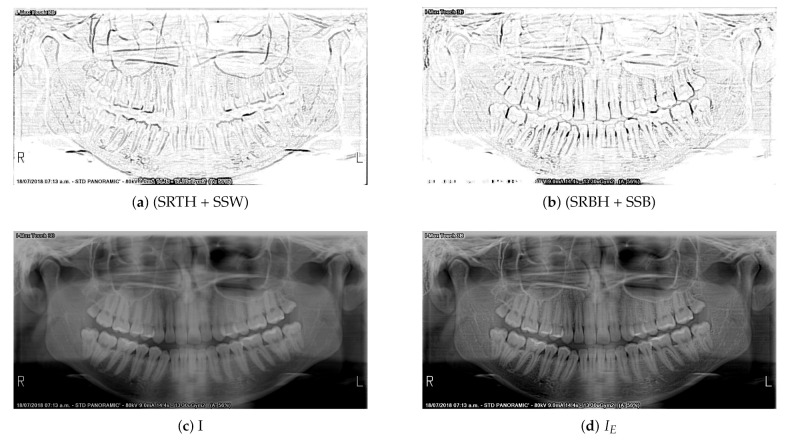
Enhancement of panoramic radiograph 34.jpg.
